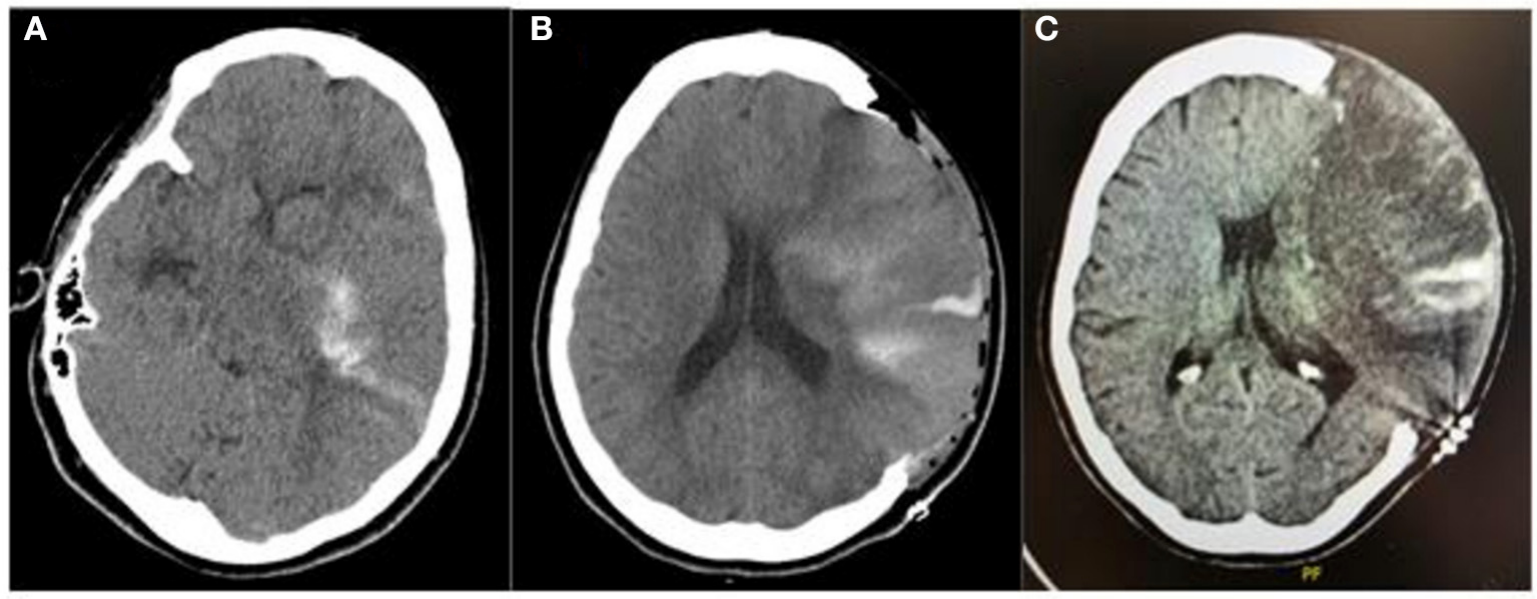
Pre- and post-craniectomy images: (A) Head CT scan at admission prior to surgery with left MCA and ICA acute ischemic stroke showing hemorrhagic transformation (PH-1); (B) Day 1 postoperative CT scan showing improvement in midline shift and cerebral edema with foci of hemorrhagic transformation, which remained stable; (C) Day 10 postoperative control CT scan.
